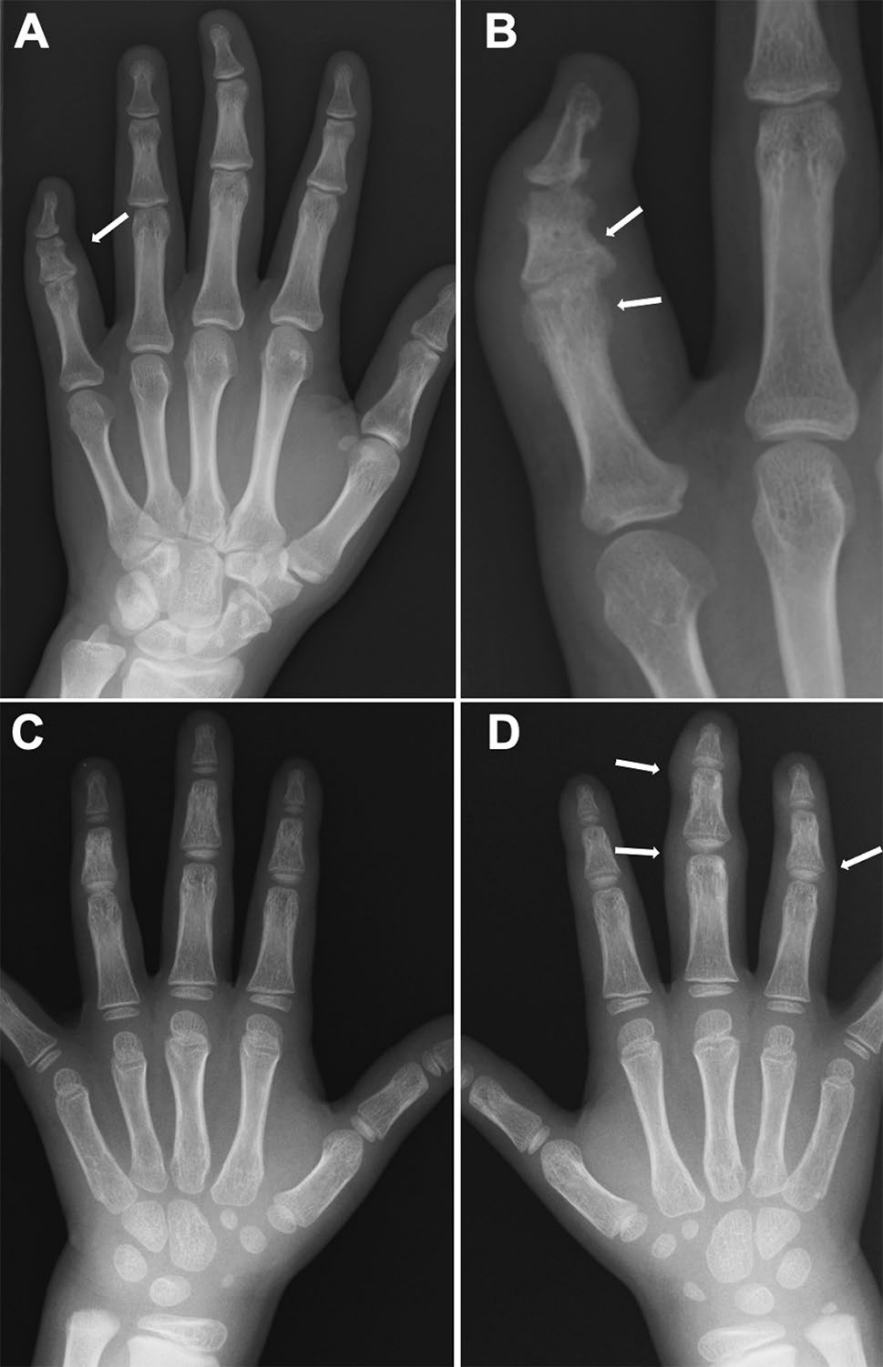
Two cases with sausage fingers with or without dactylitis. A–B. A 15-year-old girl with psoriatic arthritis, who initially presented with swelling of the left 5th finger and developed psoriasis 2 years later. The frontal radiographs of the hands showed sausage-like soft tissue swelling, joint space narrowing of the PIP, and dactylitis of the middle phalanx of the left fifth finger (arrow, A): dactylitis was depicted as moth-eaten type-trabecular derangement (arrows, B). C-D. A 5-year-old girl with oligoarticular JIA. The frontal radiographs of the hands showed sausage-like swelling of the right 3rd and 4th fingers (arrows, D); carpal ossification of the right wrist is accelerated as compared with that of the left (C). Courtesy of Professor T Hasegawa, Department of Pediatrics, Keio University School of Medicine
